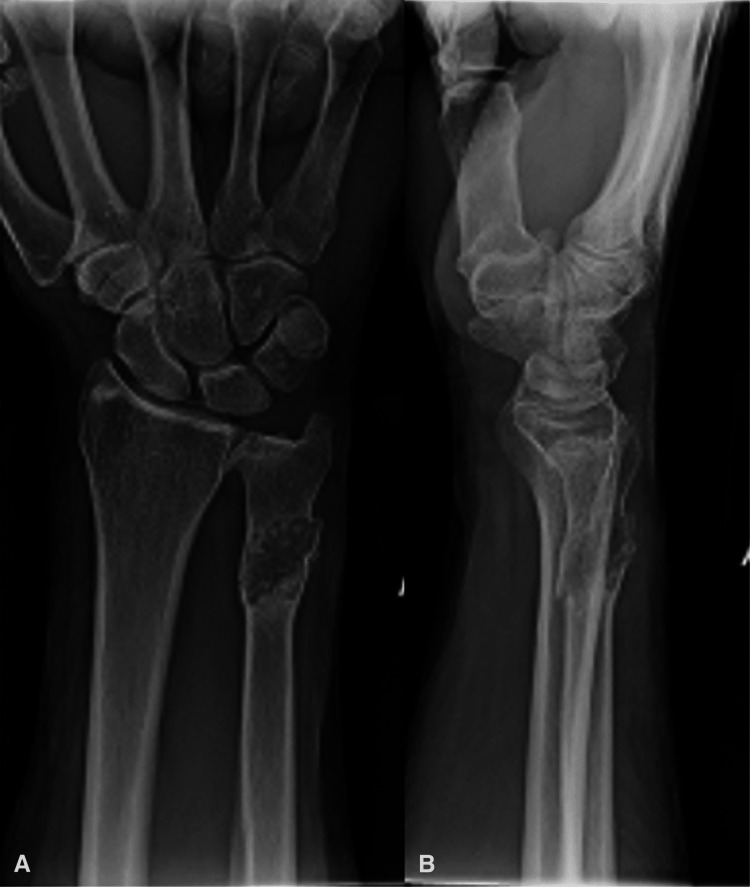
Pre-operative X-ray of the right forearm. Frontal (A) and lateral (B) view. Shows a comminuted, pathologic fracture of the right distal ulna through a lucent lesion with matrix mineralization.
